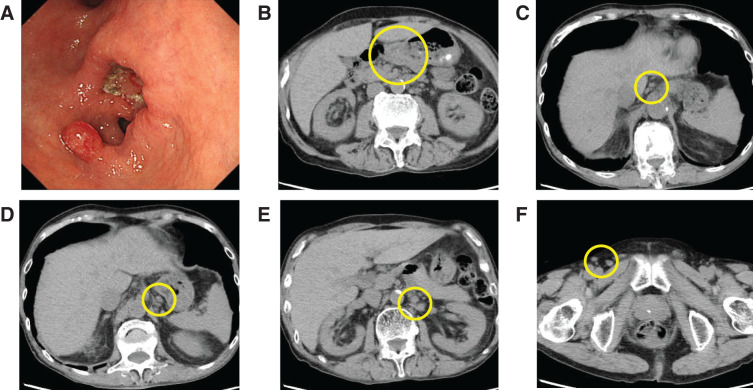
(A) Upper gastrointestinal endoscopy showing a circumferential Borrmann type 2 lesion along the lesser curvature of the gastric antrum. (B–F) Plain computed tomography images showing mild wall thickening in the gastric antrum and mildly enlarged lymph nodes (≤10 mm) along the lesser curvature and around the left gastric artery, as well as systemic lymphadenopathy including the para-aortic and inguinal regions.
